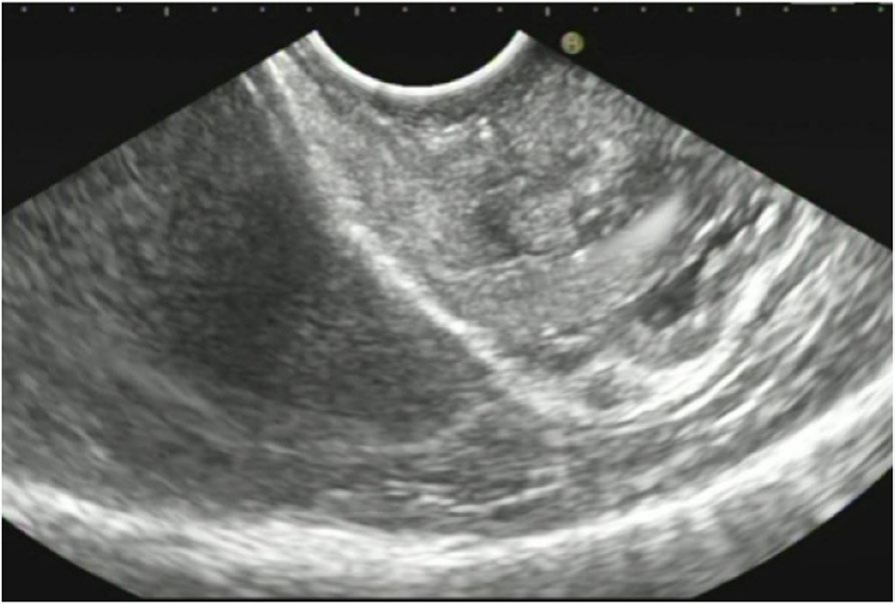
Endoscopic ultrasound (radial scan, 10 MHz) revealed the GHIP as a heterogeneous tumor with multiple small hypoechoic or anechoic areas in the third layer of the gastric wall
